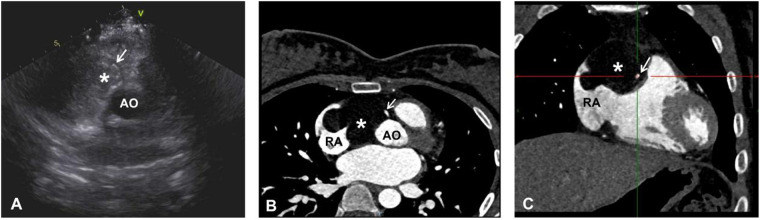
Multimodality imaging of the pericardial mass. (A) Transthoracic echocardiography (parasternal short-axis view) showing a hypoechoic mass (*) above the aortic root (AO), with the right coronary artery (arrow) coursing through it. (B) Contrast-enhanced cardiac CT (axial view) demonstrating an irregular, fat-density mass (*) within the pericardium extending toward the right atrium (RA), along with the right coronary artery (arrow) and aorta (AO). (C) Contrast-enhanced cardiac CT (coronal MPR reconstruction) showing the mass (*) in relation to the right atrium (RA) and right coronary artery (arrow).
